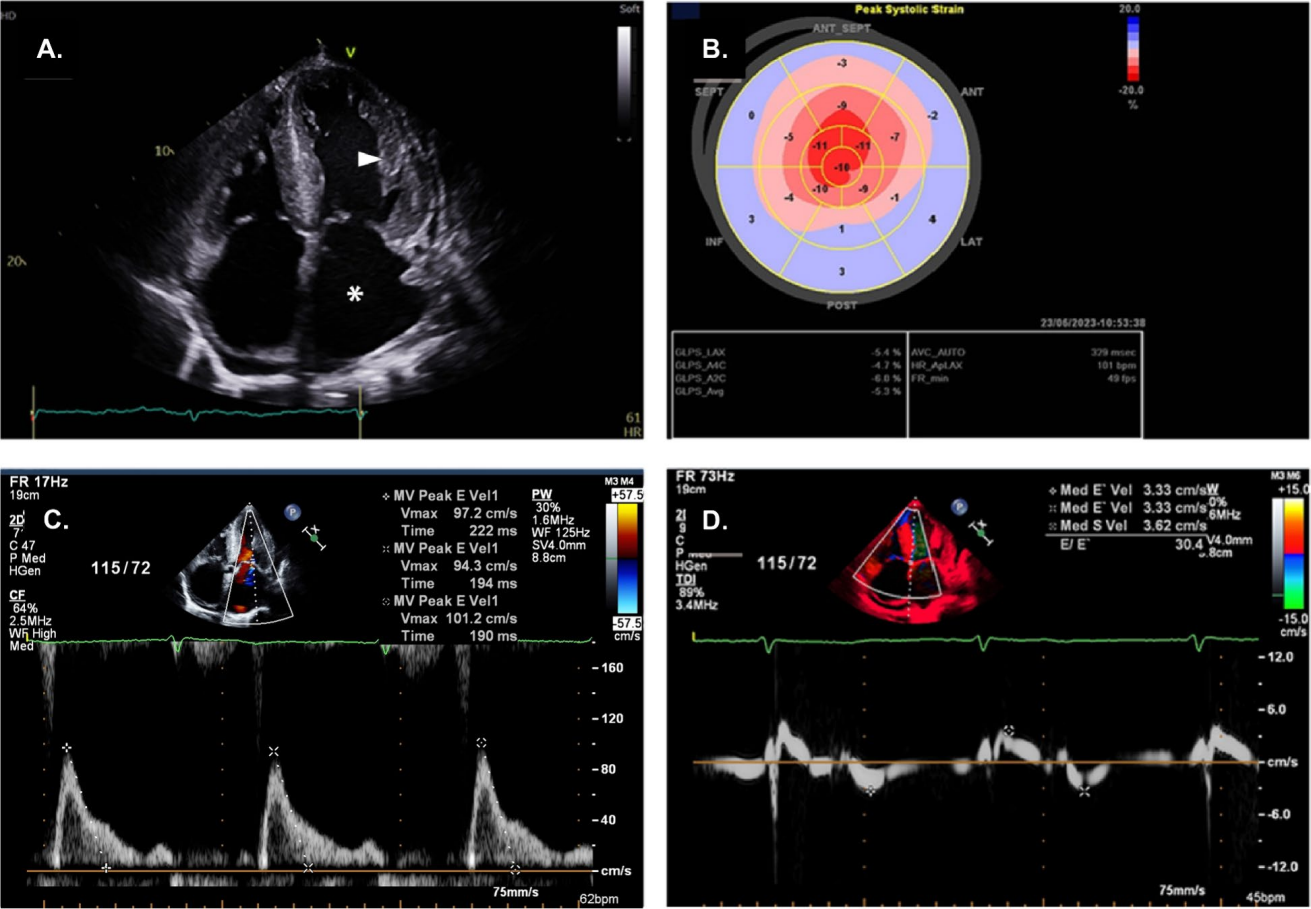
A Transthoracic echocardiography (TTE) reveals enlargement of the left ventricle and left atrium (white asterisk), increased LV diameter and a thickened LV wall with a granular sparkling texture of the myocardium (white arrowhead), and pericardial effusion. B The longitudinal strain is globally reduced on the bull’s eye plot with relative apical sparing. C Transmitral flow evaluation reveals a restrictive pattern with a high mitral inflow velocity of 100 cm/s and D an E/e′ ratio of 29
